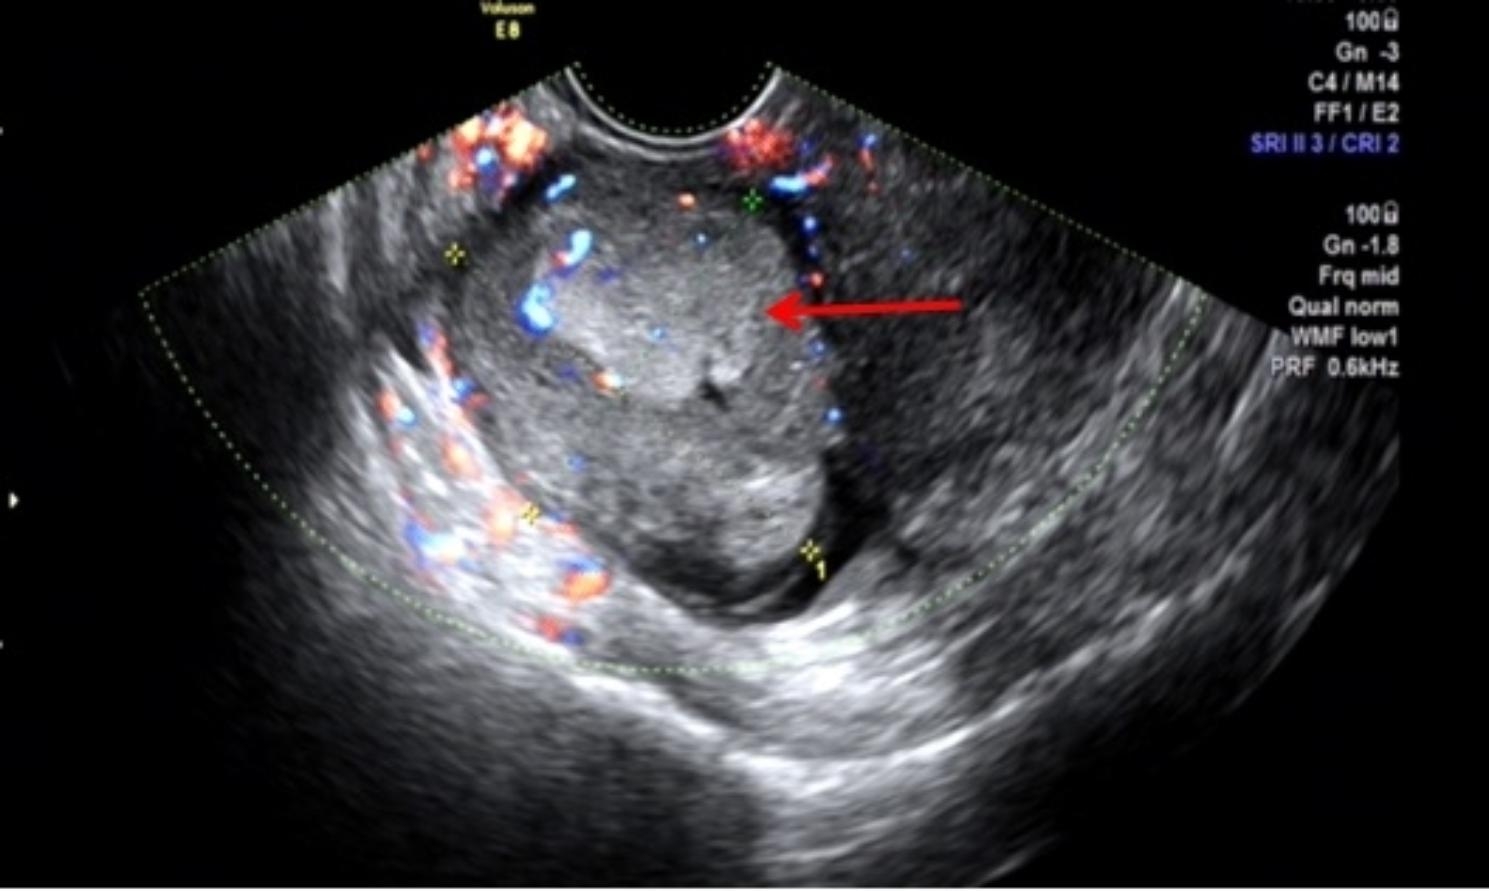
Transvaginal ultrasound showed an uneven high echo (red arrow) with a size was 48*50*39 mm in the right anterior myometrium
